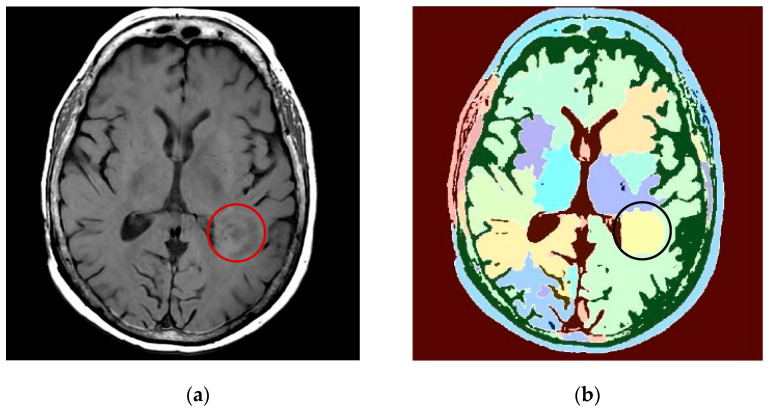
MRI of tumor lesion denoising. (a) MRI of tumor lesion denoising; (b) area recognition after MRI denoising.
